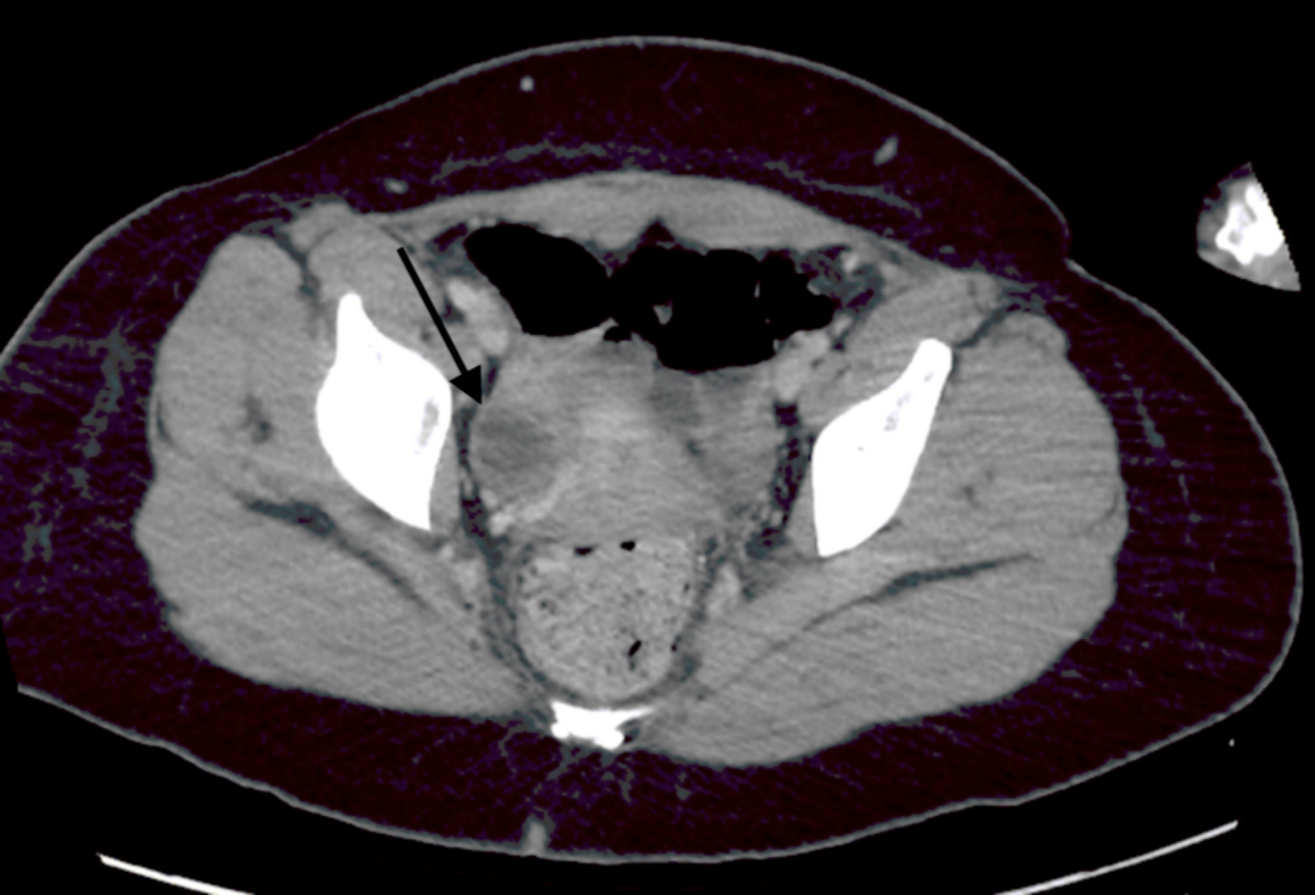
Axial CT Chest Abdomen and Pelvis with IV Contrast
